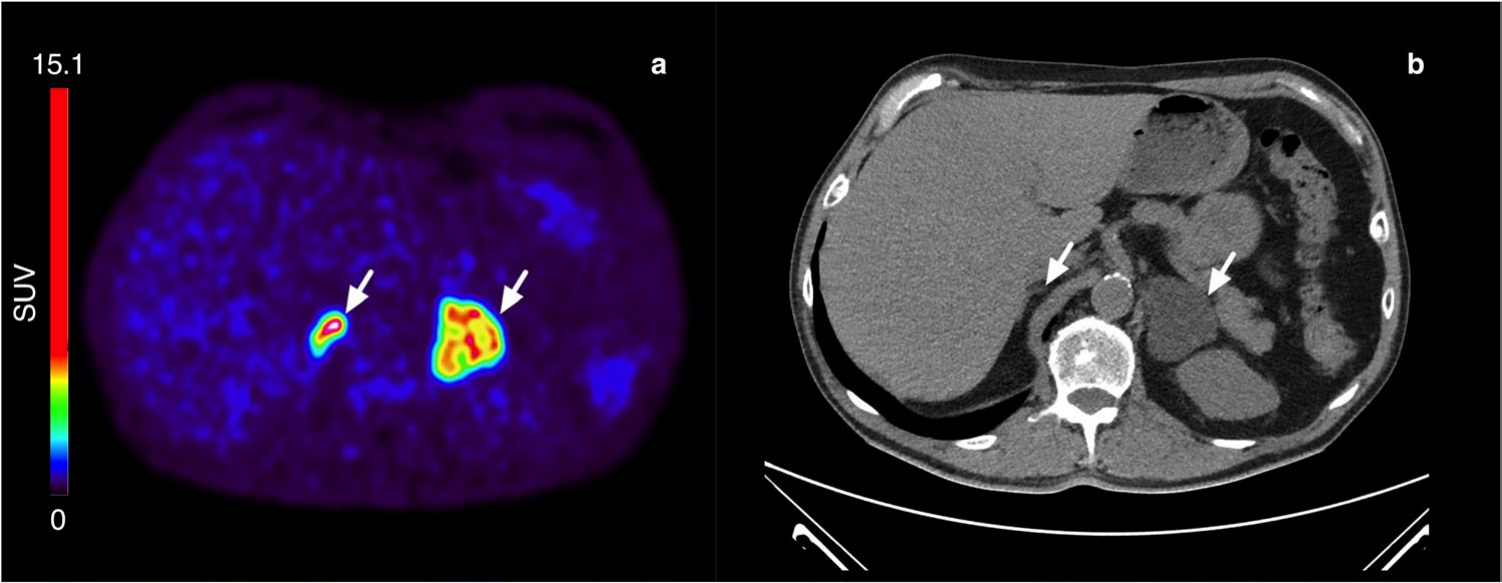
Bilateral nonfunctional adenomas(arrows), patient 15. [^18^F]CETO PET 3 h post injection (a) and CT (b), where CT was performed 17 days before [^18^F]CETO PET
